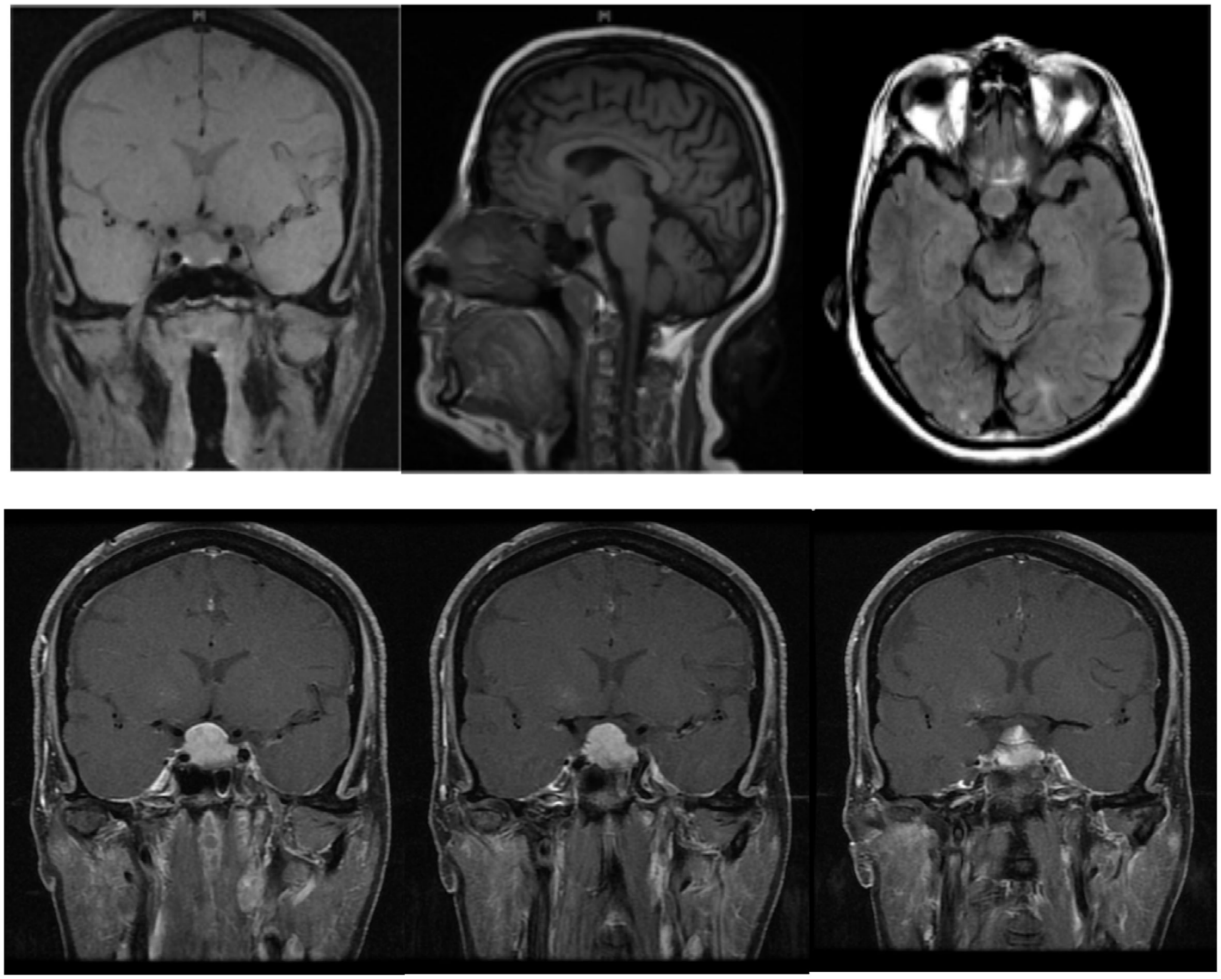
Diffuse pituitary enlargement of 1.9 cm × 1.6 cm × 1.8 cm in size is noted in pre-treatment MRI images; post-contrast images show diffuse enhancement, as well as a mass reaching up to right cavernous sinus and elevating to the optic chiasm. In the last image, a thickened pituitary stalk is seen, which deviates from the midline.
